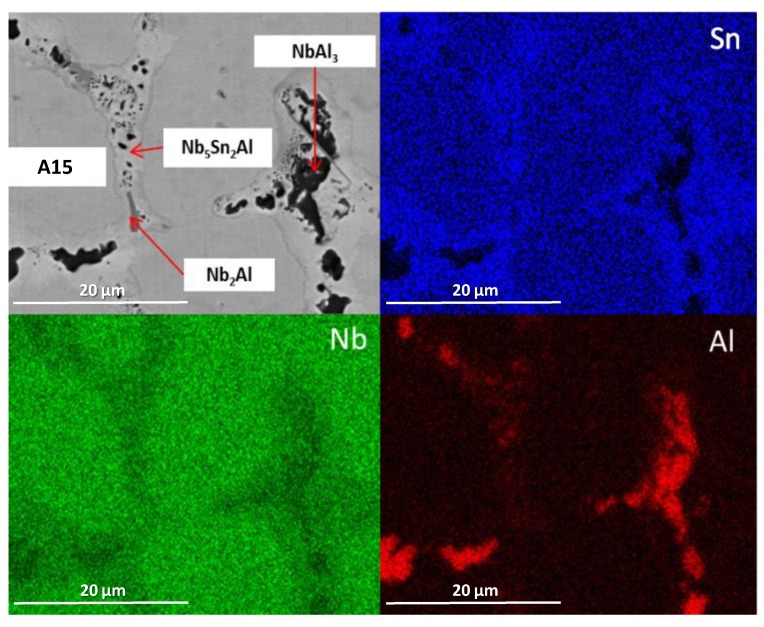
BSE image and X-ray element maps of IP4-HT 900 °C/100 h (for colour, see online version).
